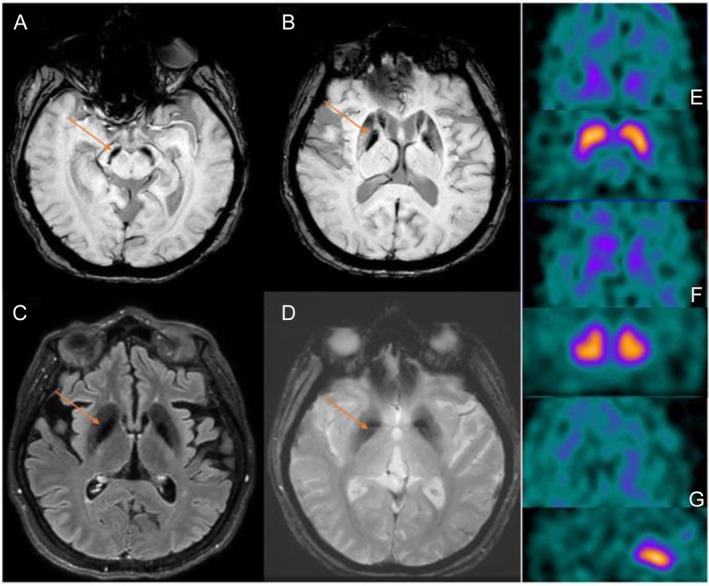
Axial magnetic resonance imaging shows bilateral hypointensity of the substantia nigra (orange arrow on a) and globus pallidus (orange arrow on b and c) on SWI (A, B) and Flair (C); and isointense linear signal of the medial medullary lamina on T2*(orange arrow on d). Ioflupane scintigraphy shows severe bilateral dopaminergic denervation (e. coronal f. axial g. sagittal; each image being followed just below by the normal image of a control case).
